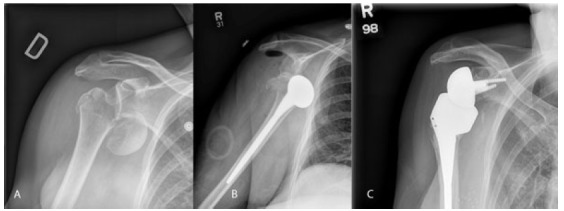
A) plain xrays of comminuted acute proximal humerus fracture-dislocation. Note comminution of tuberosities and humeral head displacement with minimal calcar remaining. B) AP of post-operative day one humeral head replacement showing dislocation of prosthesis from glenoid. C) Six month post-operative follow-up AP imaging following revision to reverse total shoulder arthroplasty. Patient was doing functionally well with no complaints of pain and range of motion continuing to improve.
